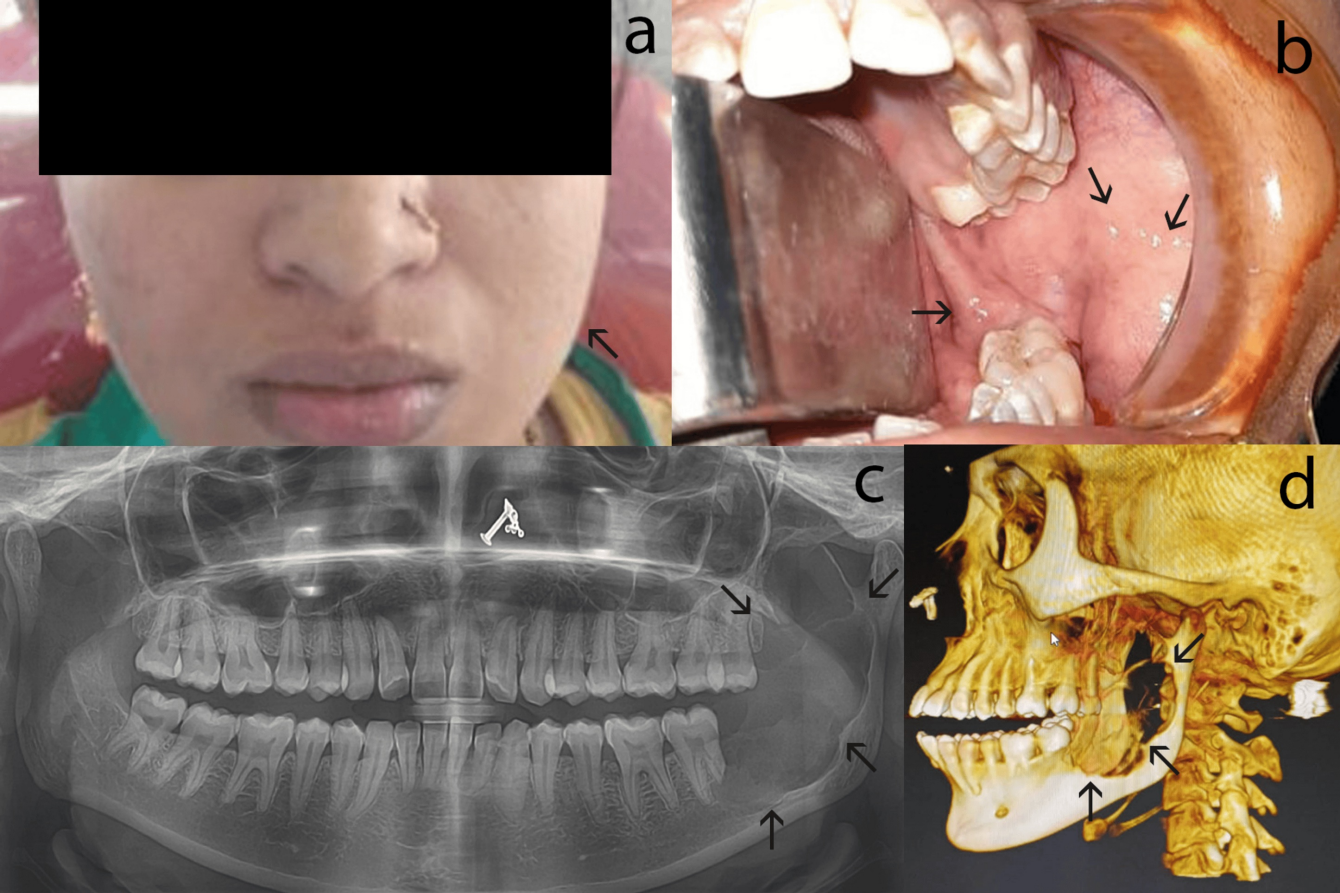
Clinical and radiographic findings of this case (a) An extraoral photograph showing a slight swelling in the mandibular left posterior region. (b) An intraoral photograph displaying the affected region. (c) An OPG revealing multilocular radiolucency that extends from tooth 37 to the ramus. (d) A 3D CBCT reconstruction showing the involvement of the left mandible and ramus of the mandible (arrows pointing to the lesion). OPG: orthopantomograph; CBCT: cone-beam computed tomography
